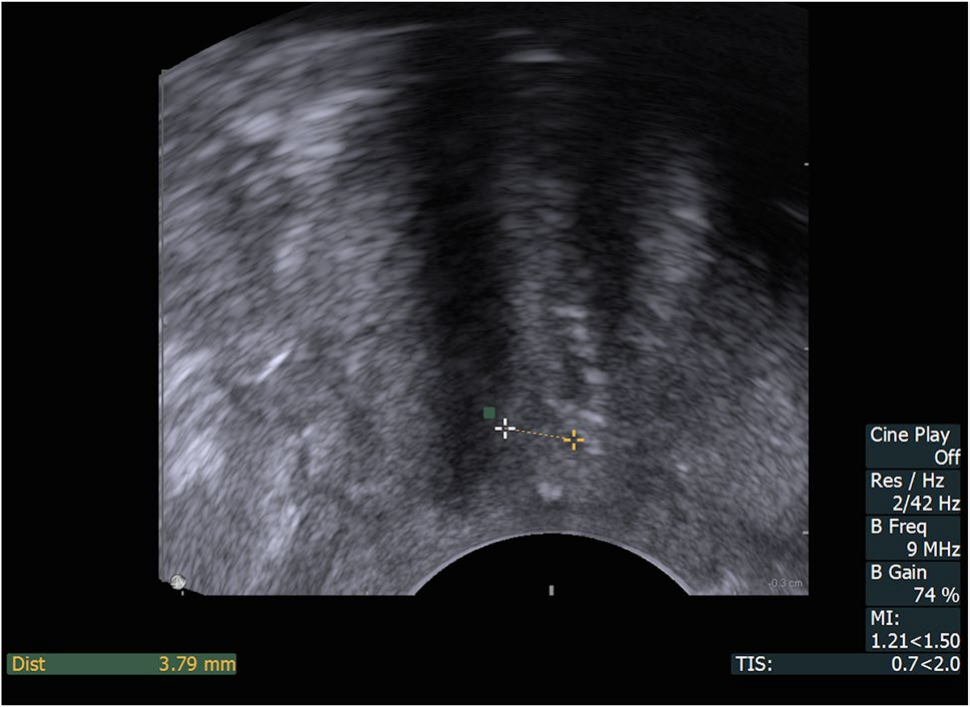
Ultrasonographic tape visualisation in mid-sagittal scan: Dist: distance between the lower edge of the tape and the hypoechogenic urethral complex (T_UC_)
